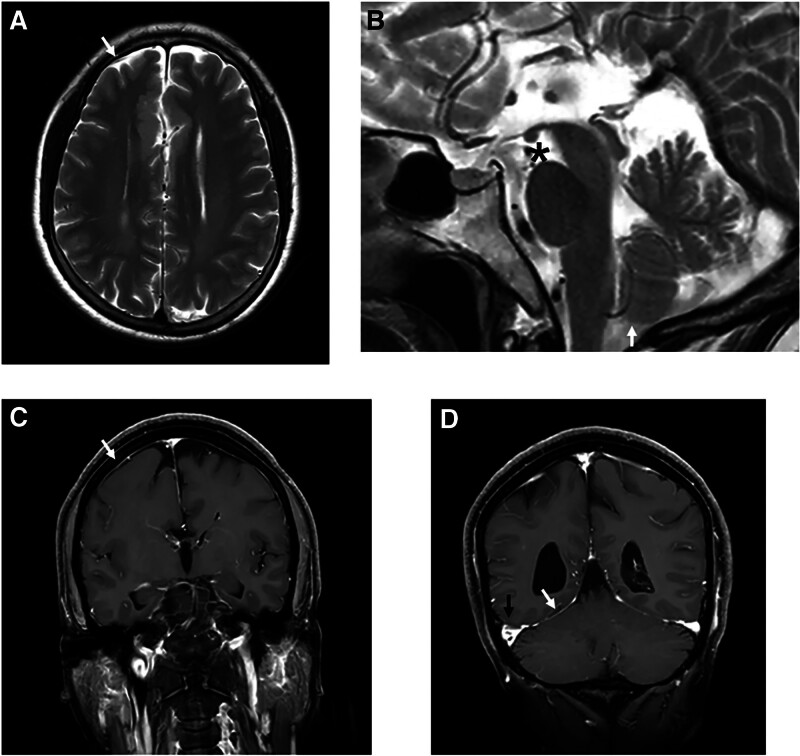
Imaging findings of spontaneous intracranial hypotension. (A) Axial noncontrast T2-weighted image with subdural effusion (white arrowhead). (B) Sagittal noncontrast T2-weighted image with decreased mamillopontine distance (asterisk: 5.43 mm, pathologic ≤ 6.5 mm) and low-lying cerebellar tonsils (white arrowhead). (C, D) Coronal contrast-enhanced T1-weighted image with slightly dural enhancement (white arrowheads) and venous engorgement (black arrowhead).
